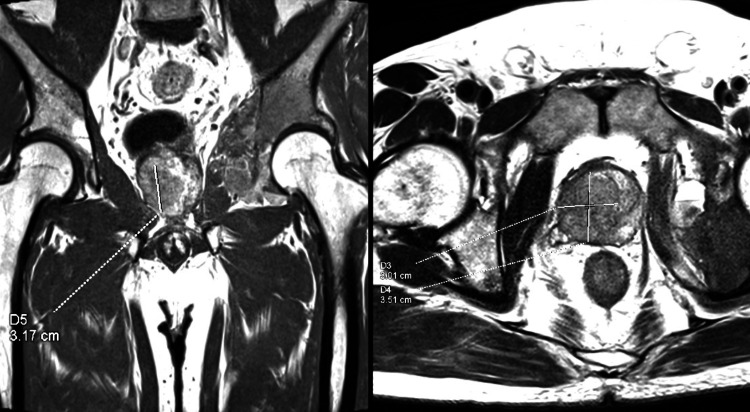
MRI of the left hip Hip MRI identified a T2W hypointense mass (3.01x3.51x3.17cm) with marked restricted diffusion that is noted at the prostate from base to apex, with the epicenter on the right side and extension to the left side, highly suggestive of prostate metastasis.
